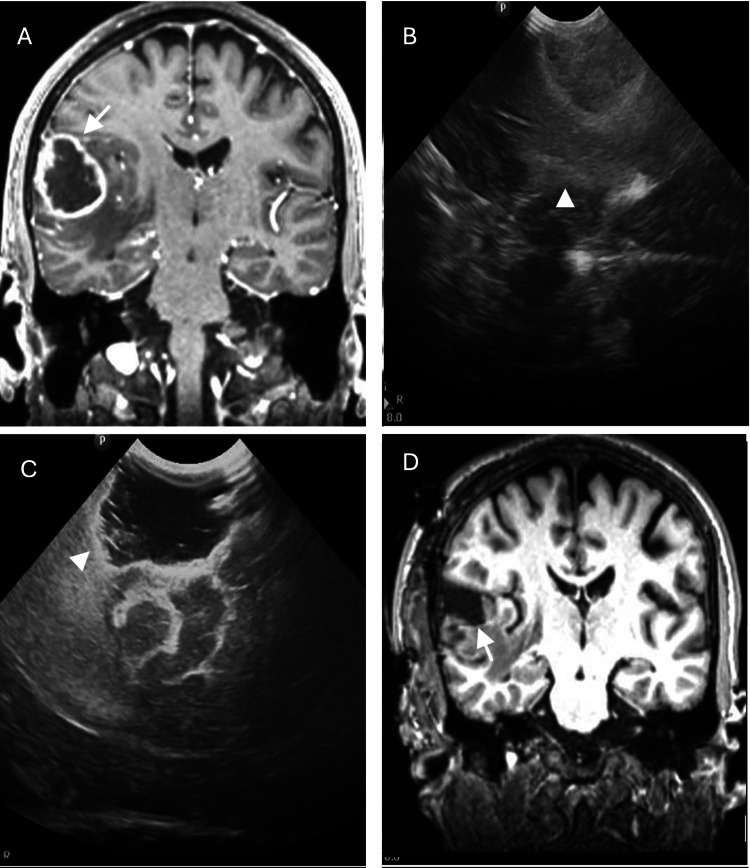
Right temporal high-grade glioma (A) Pre-operative T1 gadolinium-enhanced MRI shows intra-axial tumor with contrast enhancement in the periphery and perilesional edema (white arrow). (B) Intraoperative ultrasound shows intra-axial tumor with central hypoechoic and hyperechoic portions in the periphery, the edges are well defined (white arrowhead). (C) Post-operative intraoperative ultrasound image shows total resection of tumor (white arrowhead). (D) Post-operative T1 gadolinium-enhanced MRI shows total resection of tumor (white arrow).
